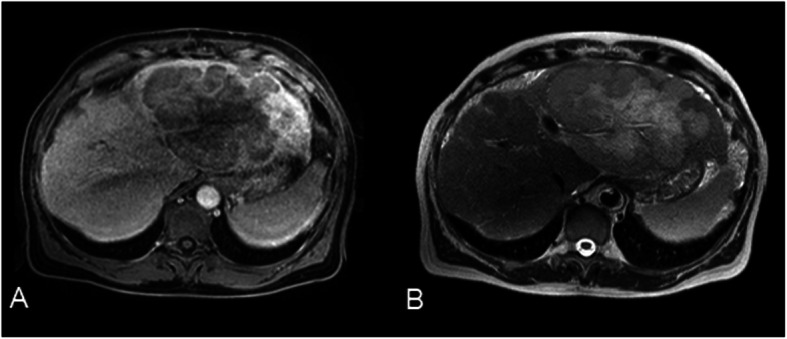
Abdominal magnetic resonance imaging revealing a nodular lesion in the left lobe measuring 15.6 × 10.9 cm, with isointense signals in both T1 (a) and T2 (b) and heterogeneous enhancement after contrast administration, with necrosis in the central area
